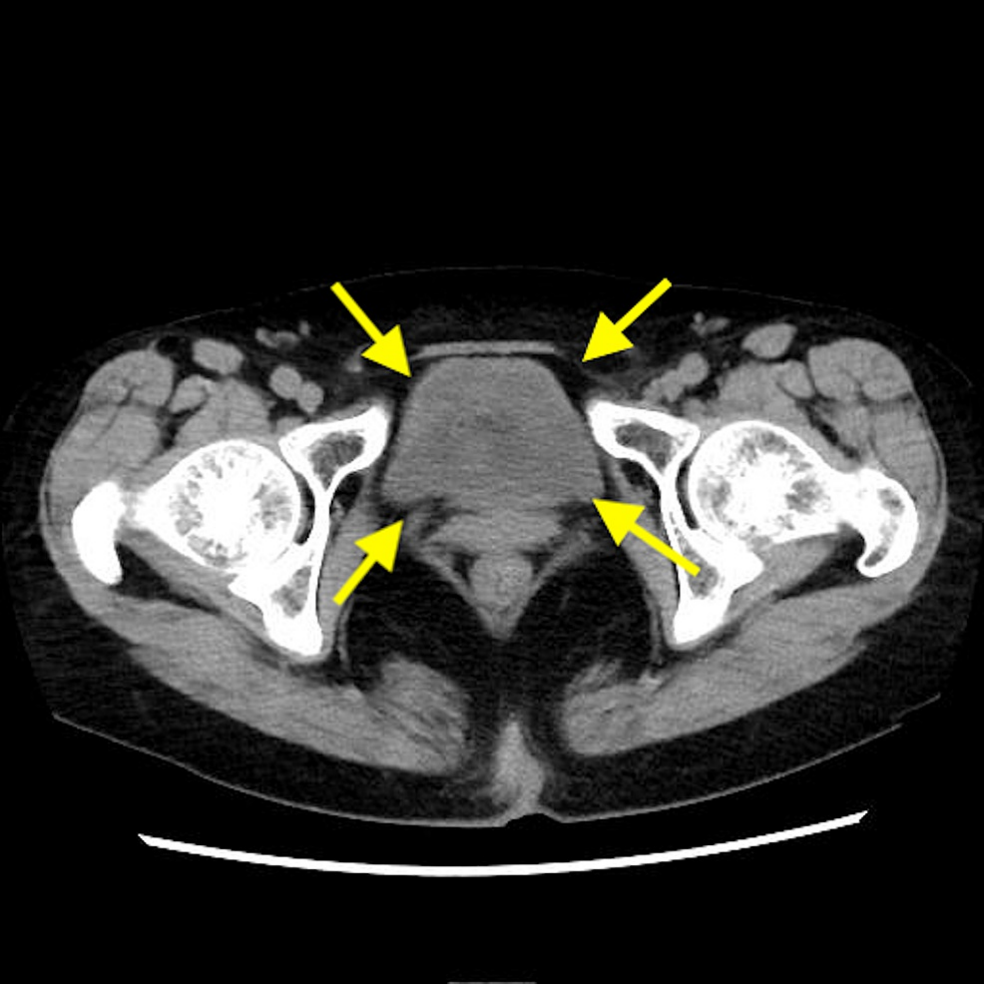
Computed tomography Yellow arrow: bladder
